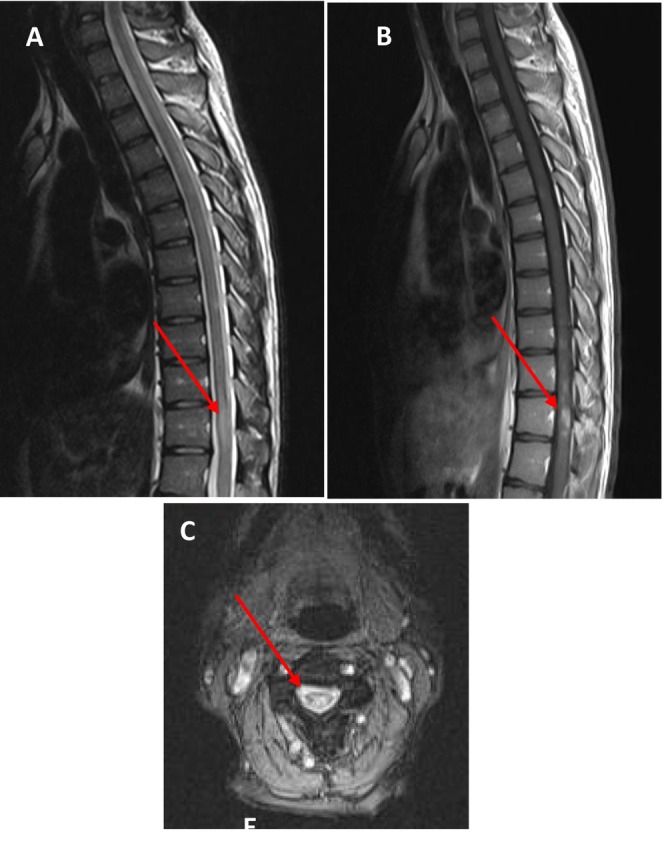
(A) Sagittal T2‐weighted MRI of the thoracic spine without contrast: The image shows a hyperintense lesion. (B) Sagittal T1‐weighted MRI of the thoracic spine with contrast: The image demonstrates an enhanced lesion. (C) Axial T2‐weighted MRI of the spine: The image shows a hyperintense lesion in the central cord of thoracic level.
